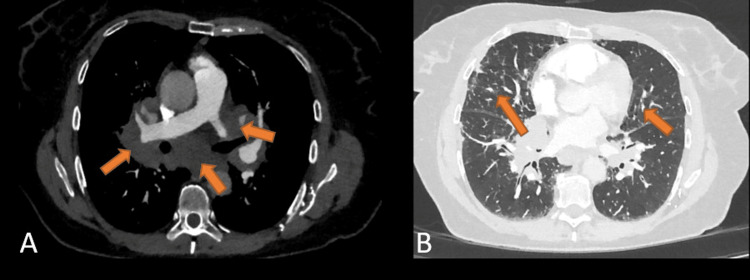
Initial CT of the thorax (axial view). A. Increased, enlarged, and confluent hilomediastinal lymph nodes (arrows). B. Bronchial thickening and interlobular septal thickening accentuated in both basal lobes, with peribronchovascular consolidations (arrows).
